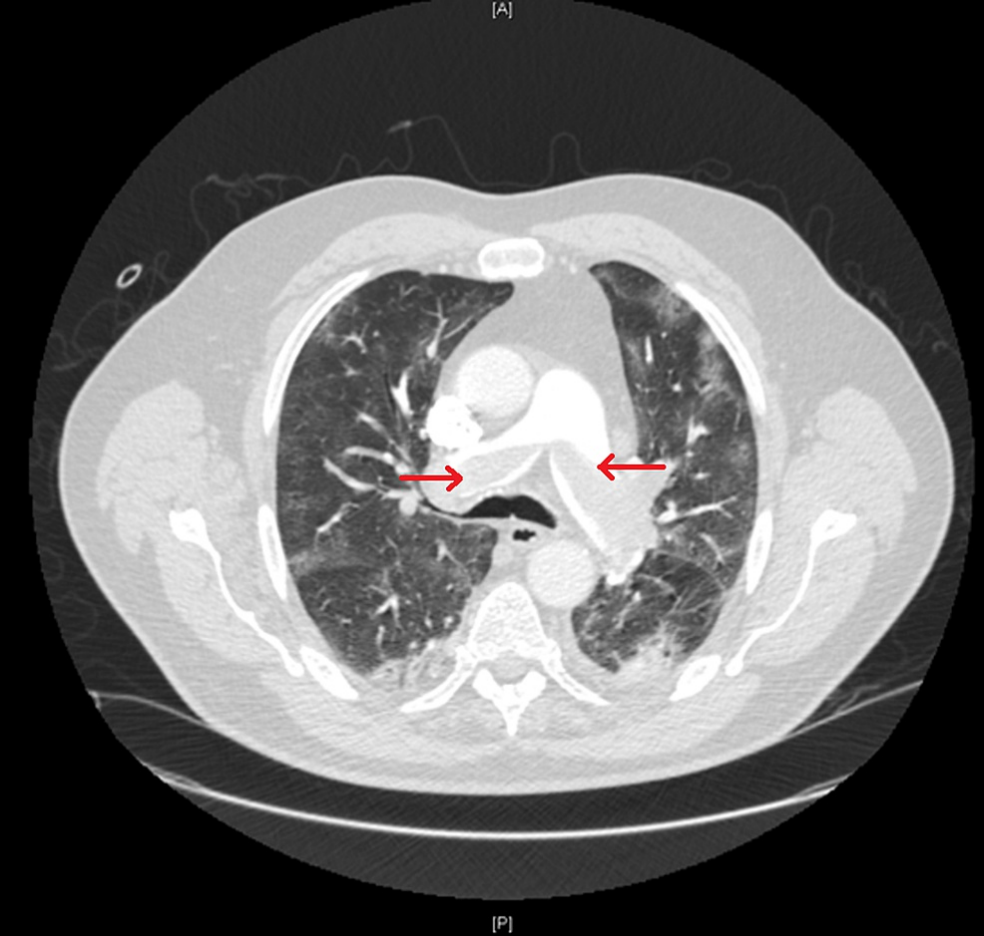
Computed tomography demonstrating saddle pulmonary embolism at the bifurcation of the pulmonary trunk (red arrows).
